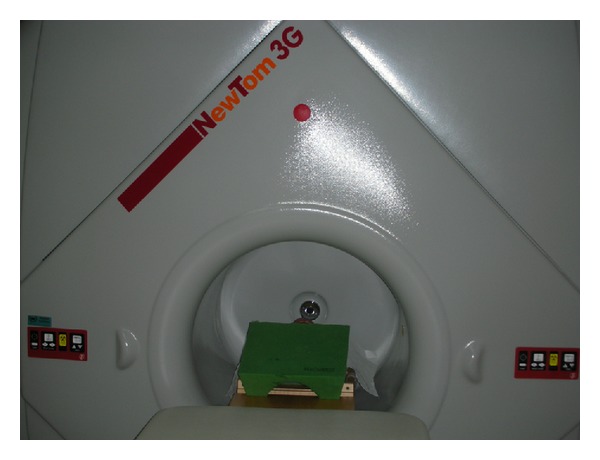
Newtom cone beam 3D imaging equipment.
